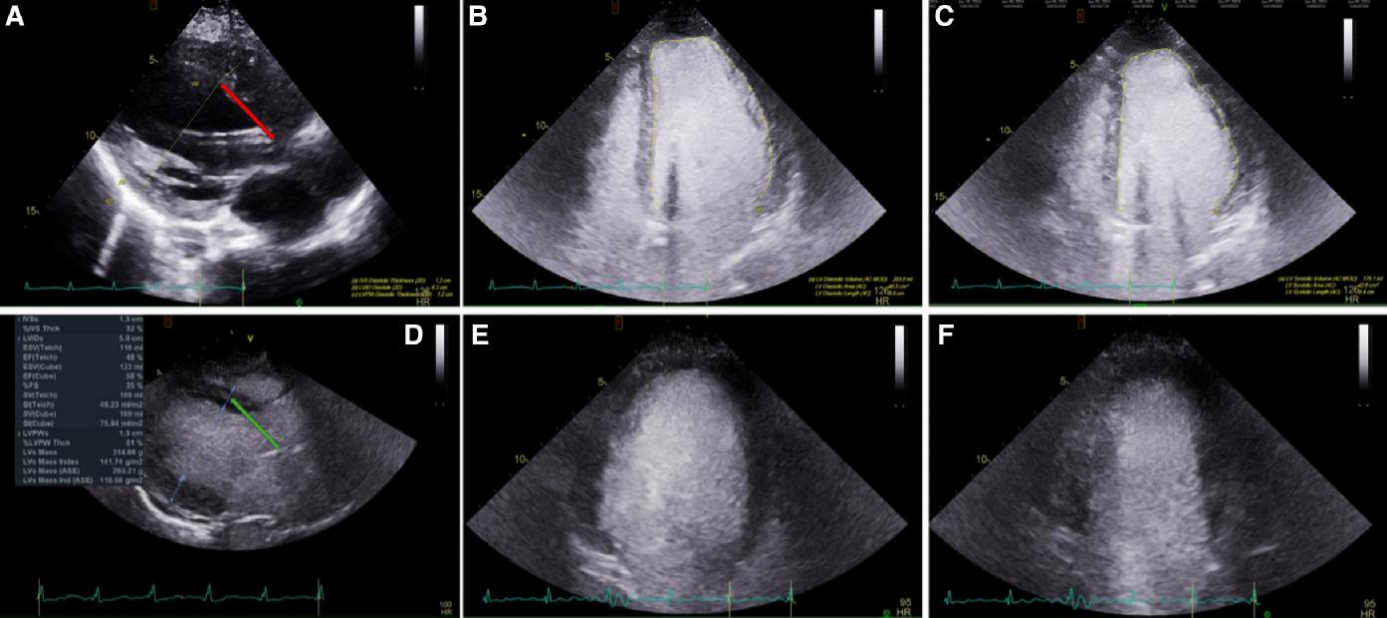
Transthoracic echocardiogram A to C, before and D to F, 2 weeks after thyroid storm treatment. A and D show changes in left ventricular (LV) inner diameter from 6.3 cm initially (A, red line) to 5.0 cm after treatment (D, green line). B and C show the initial contrast-filled LV between B, diastole and C, systole, demonstrating an ejection fraction (EF) of 15% to 20%. E and F show the posttreatment contrast-filled LV between E, diastole and F, systole, demonstrating an EF of 48%.
